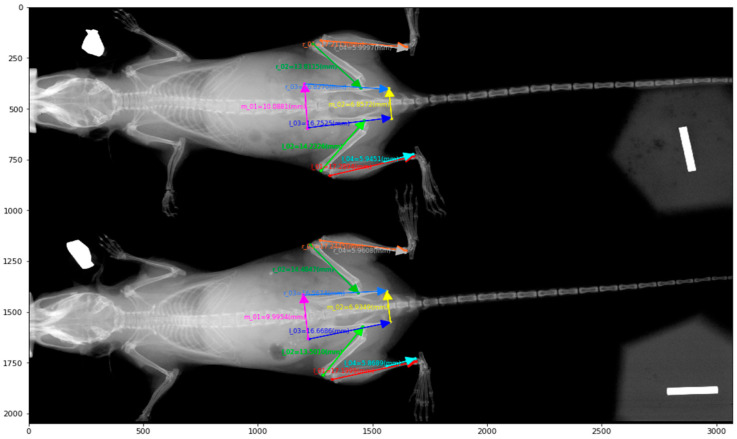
A Sample X-ray image and its annotations for bone length measurements.
